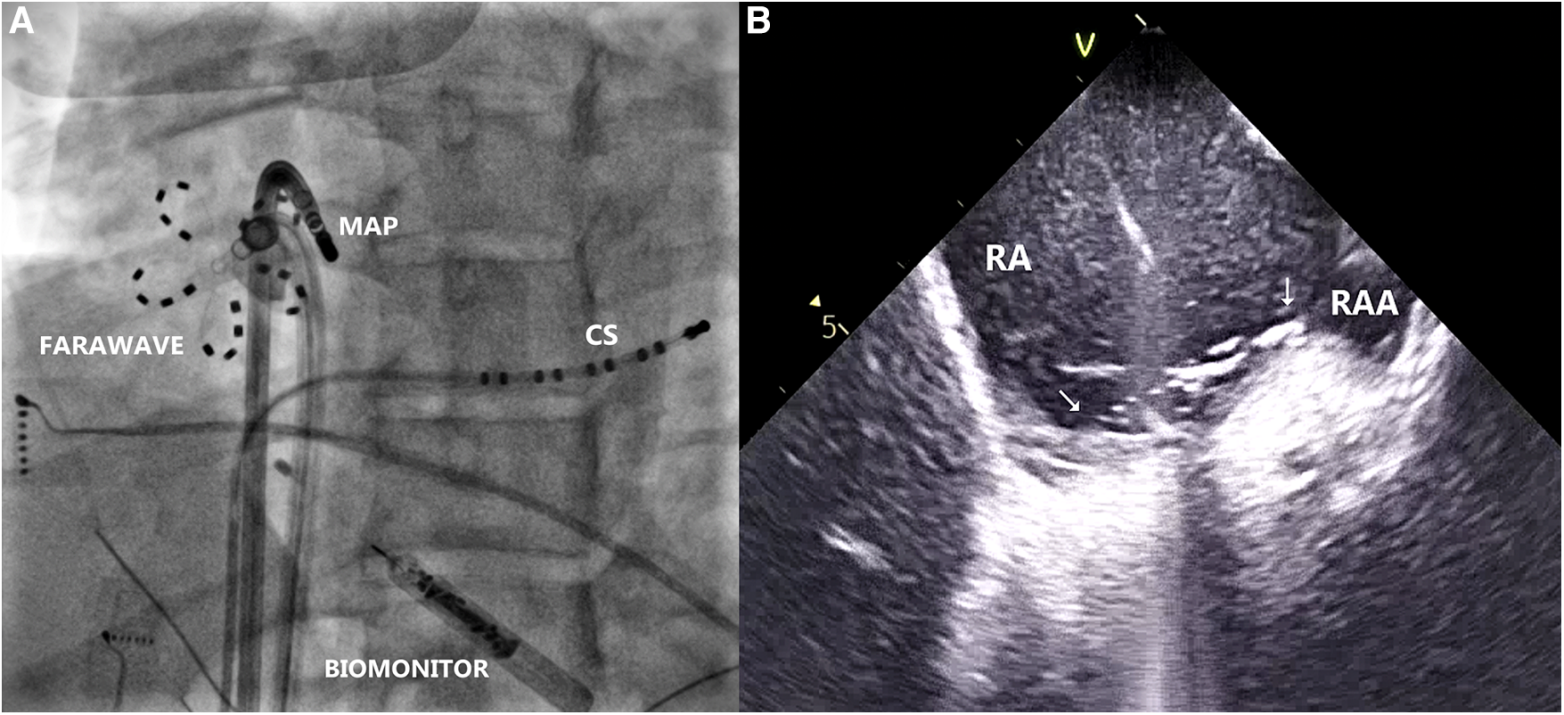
(A) Radiogram in left anterior oblique projection, showing farawave catheter at the site of the earliest atrial activation during ventricular pacing, parallel to mapping catheter (MAP). Decapolar catheter is introduced in the coronary sinus (CS). Biomonitor is visible in lower part of the image. (B) Intracardiac echocardiogram depicting location of the Farawave catheter and its contact with the tissue at the ablation site (arrows). RA, right atrium; RAA, right atrial appendage.
